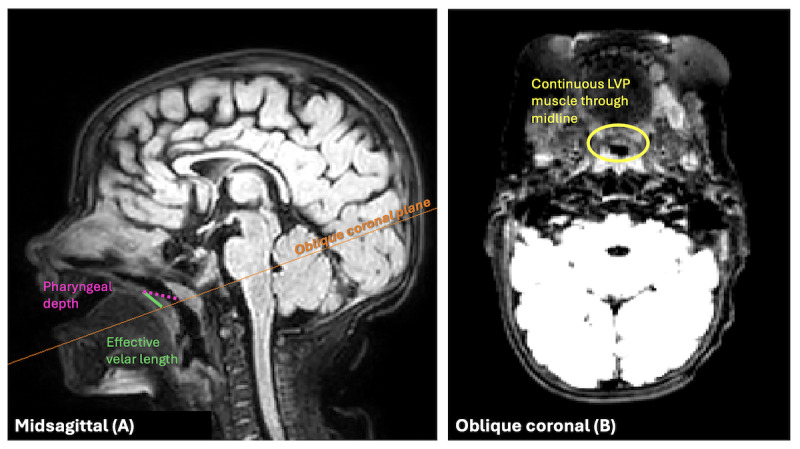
Magnetic resonance image of a typically developing toddler demonstrating the anatomy of interest when considering the risk of velopharyngeal insufficiency. LVP: levator veli palatini.
